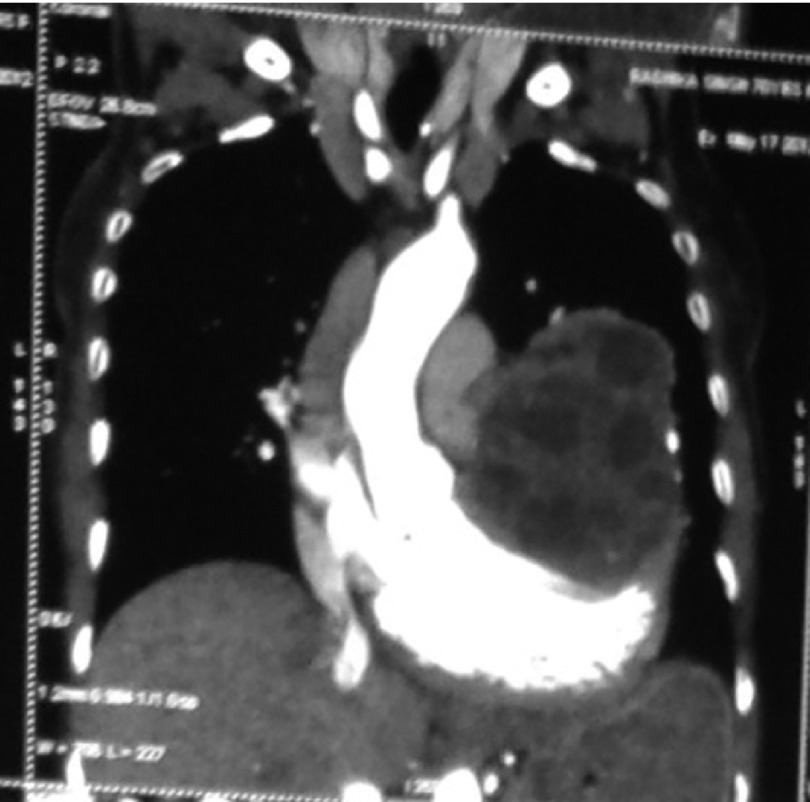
CT Scan Showing Hydatid Cyst in Pericardium
